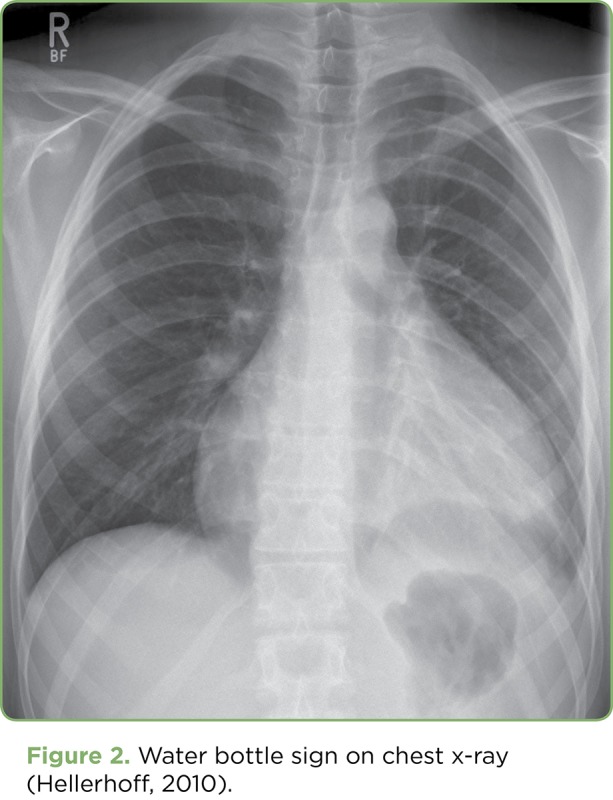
Water bottle sign on chest x-ray (Hellerhoff, 2010).
